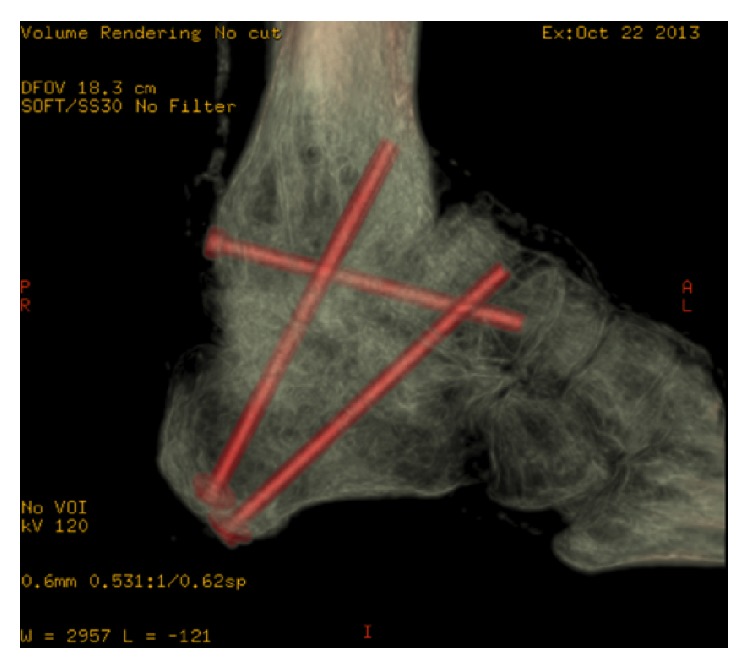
CT demonstrating subtalar fusion and some union across talar neck.
